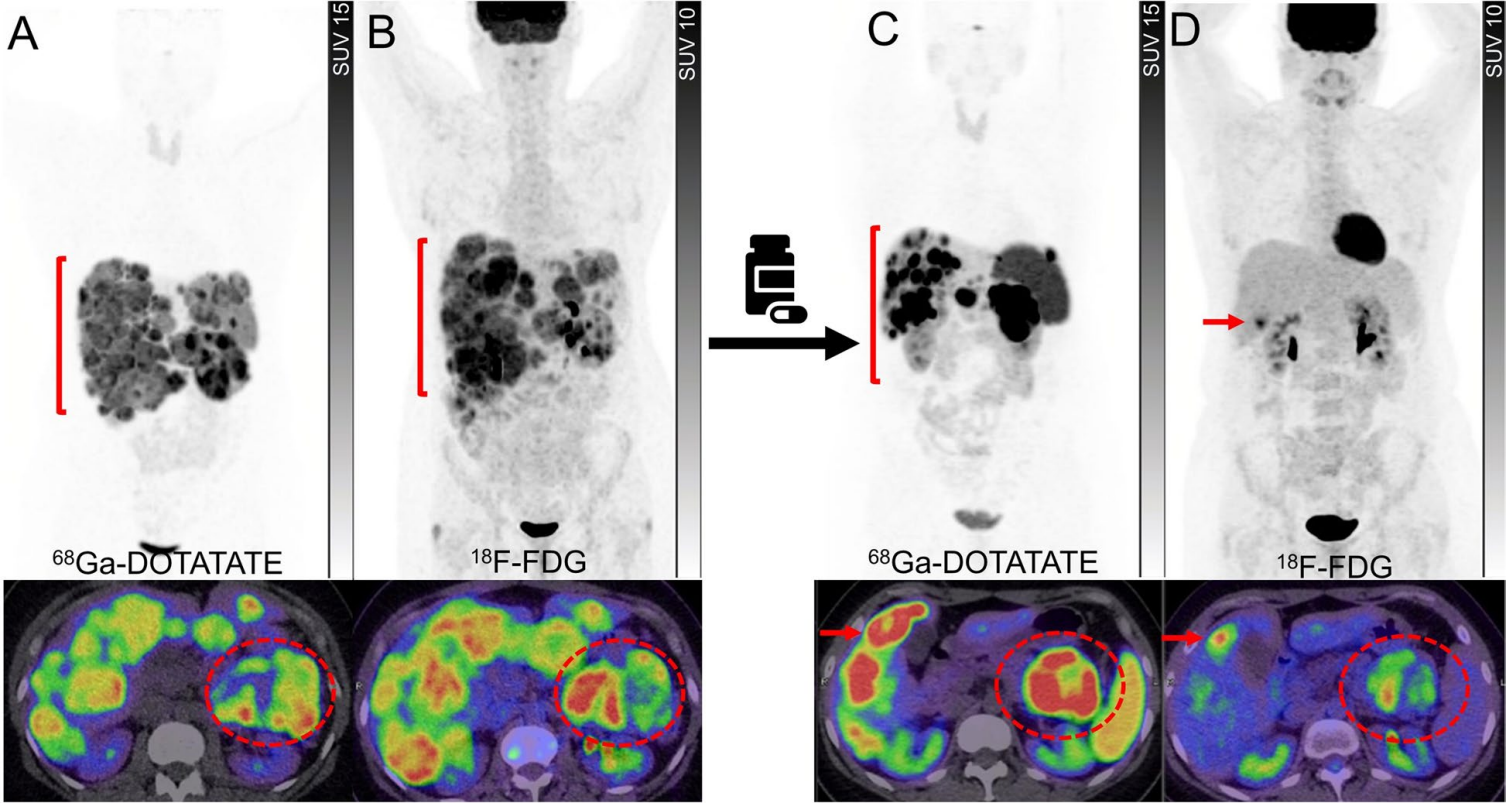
A patient with well-differentiated pancreatic NET (G3, Ki67 40%). ^68^Ga-DOTATATE PET (A) and ^18^F-FDG PET (B) showed heterogeneously tracer avid pancreatic mass with some areas (the medial component of the mass) demonstrating relatively higher ^18^F-FDG-avidity compared to ^68^Ga-DOTATATE avidity (circles). Large volume hepatic metastases were also noted with several areas demonstrating higher avidity on ^18^F-FDG PET (brackets). Due to high-grade pathology and a large-volume disease with some areas of heterogeneity on baseline scans, the patient was deemed not suitable for treatment with ^177^Lu-DOTATATE and therefore treated with chemotherapy. Restaging studies following 4 cycles of chemotherapy showed marked partial response on ^68^Ga-DOTATATE PET (C) with disease demonstrating more homogenous and higher tracer avidity (bracket). Contemporaneous ^18^F-FDG PET (D) showed marked partial response in the pancreas (circle) and resolution of metabolic activity in the liver except for one lesion which also demonstrated ^68^Ga-DOTATATE avidity (arrows). At this time point, the patient was deemed suitable for treatment with ^177^Lu-DOTATATE to consolidate the response to the prior treatment
